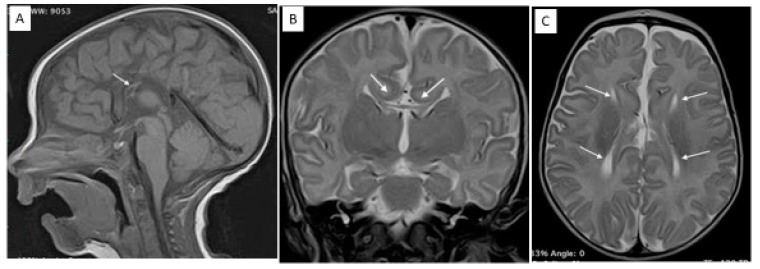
RJ’s magnetic resonance imaging. (A) Sagittal T1 WI. The corpus callosum is absent. There is a small residual portion (arrow) in the genu/anterior body. (B) Coronal turbo T2 WI. The cingulate gyri are everted as a result of the absence of the callosal fibers (arrows). There is a small residual portion of the genu/anterior body (thin arrows). (C) Axial turbo T2 WI. The walls of the lateral ventricles are dysmorphic because the Probst bundles do not cross the midline.
